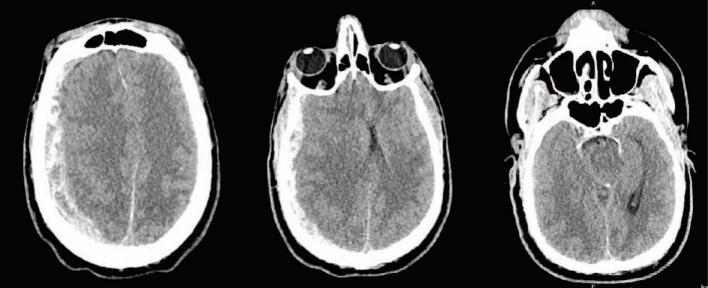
Admission CT Head. CT Head on admission demonstrated a 1.3 cm right SDH with 1.2 cm of midline shift and evidence of transtentorial herniation.
